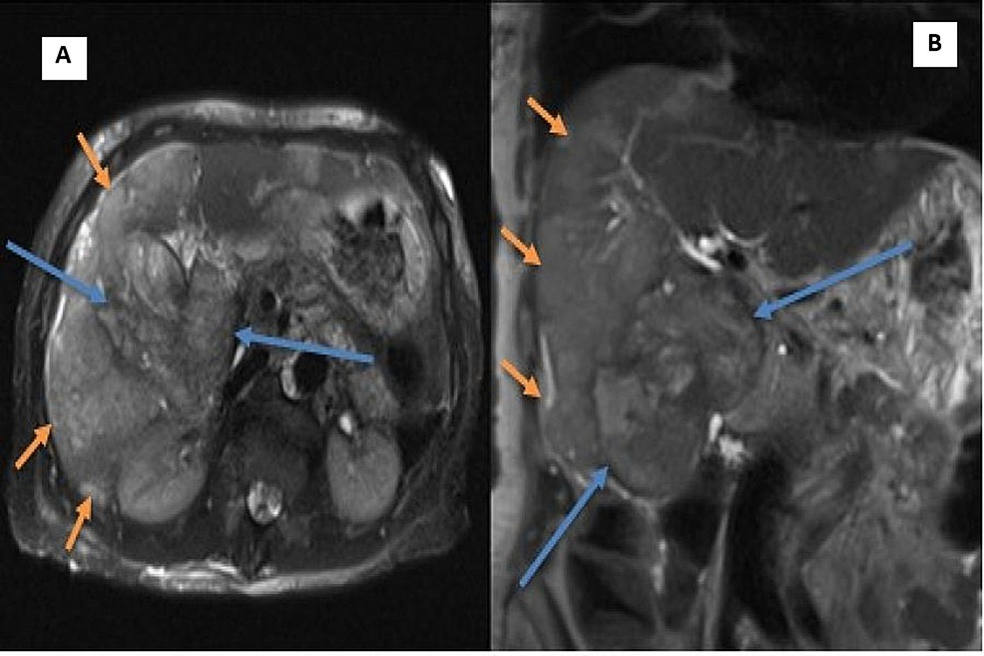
(A and B) T2 MRI images of the abdomen, axial and coronal, respectively, performed shortly after OGD, showing an extensive soft tissue within the gallbladder and its extension into the duodenum (blue arrows) and high signal within the liver from direct soft-tissue infiltration (orange arrows). MRI, Magnetic resonance imaging; OGD, oral gastroduodenoscopy.
